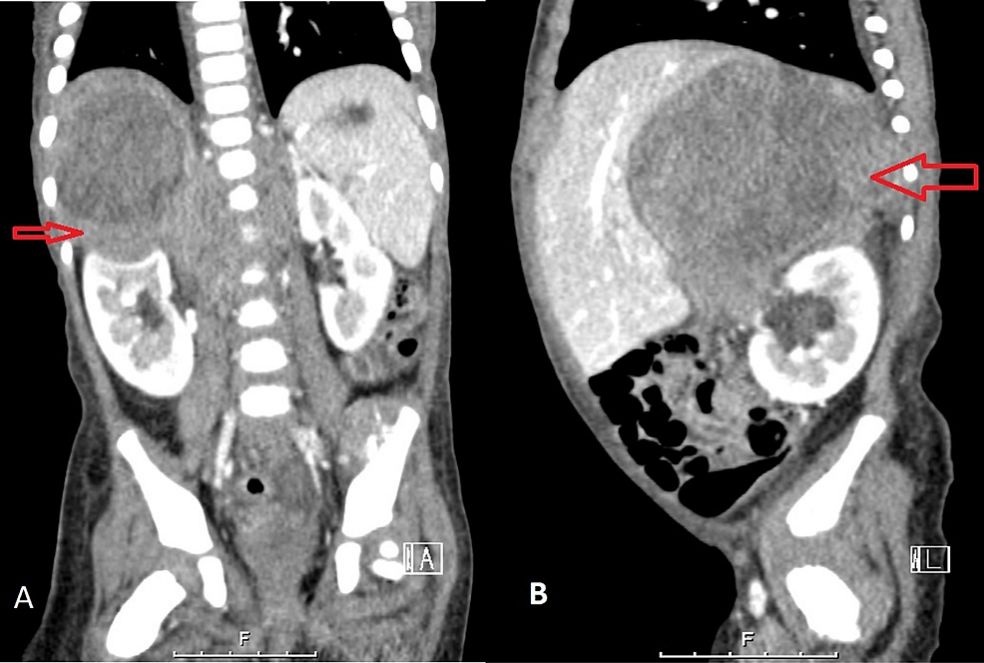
Abdominal and pelvic CT with contrast (A) coronal and (B) sagittal, showing large heterogeneously enhancing retroperitoneal mass arising from the right adrenal gland representing neuroblastoma.
